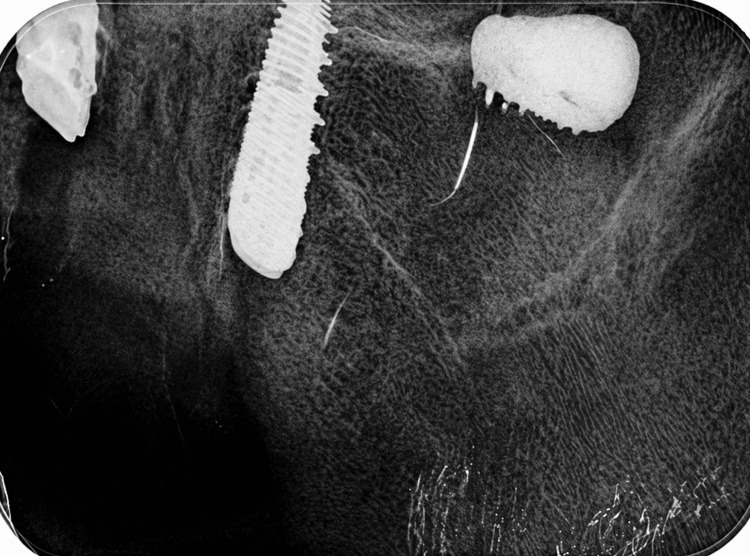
Periapical radiograph showing the dental implant displaced into the left maxillary sinus
